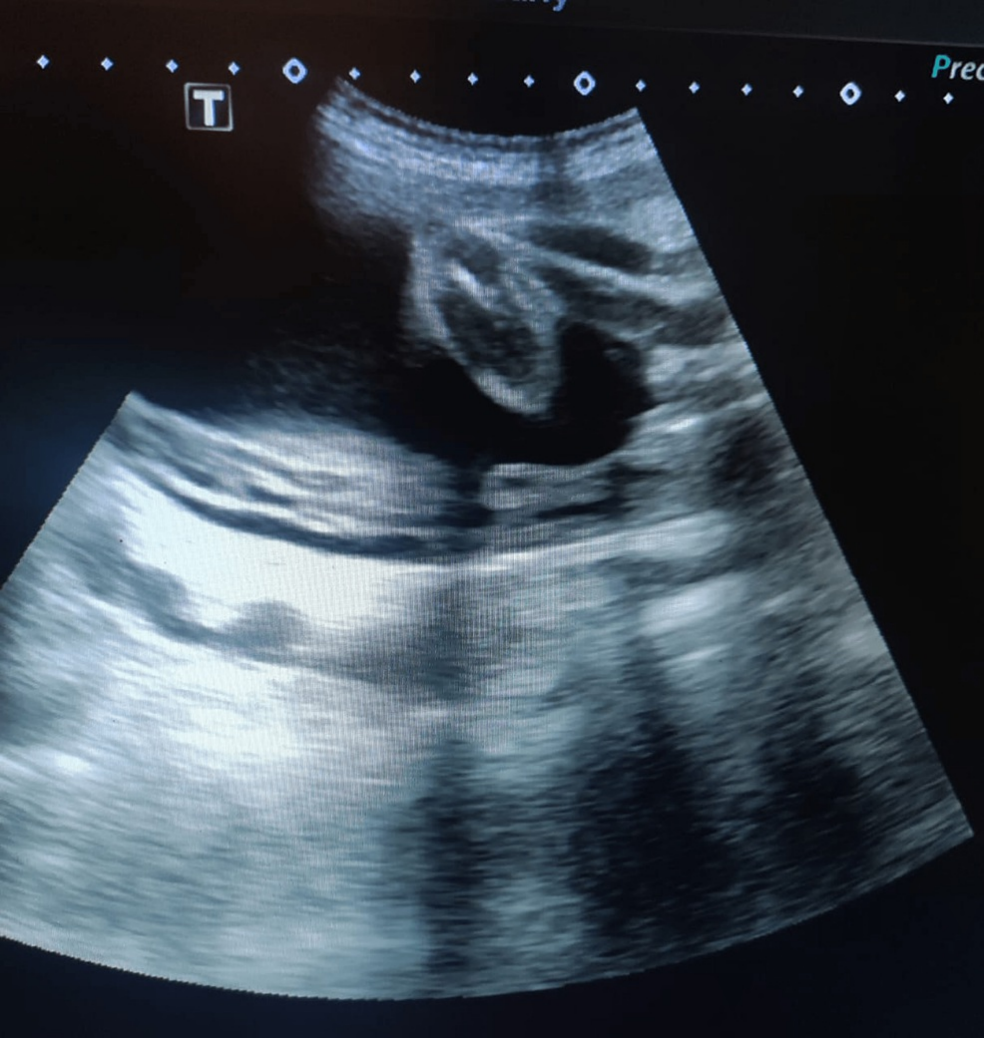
Normal fetal ultrasound at 26 weeks of gestation
